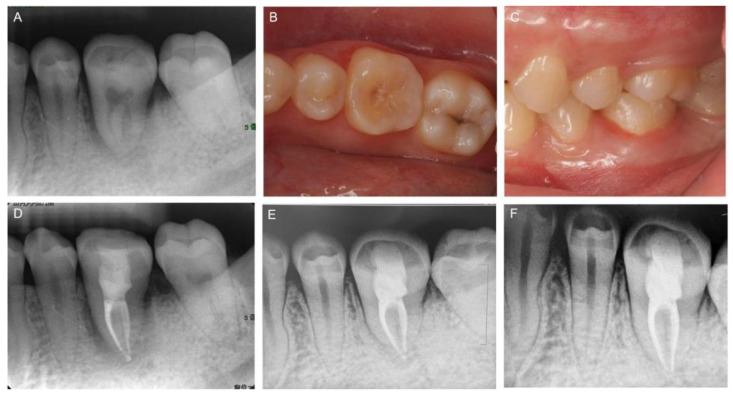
Clinical follow-up. (A) Postoperative radiograph taken immediately after autotransplantation. An occlusal view (B) and a buccal view (C) at the 3-week follow-up. (D) Endodontic treatment performed after 3 weeks. The 8-month follow-up radiograph (E) and 1-year follow-up radiograph (F) showing no signs of root resorption and the normal functioning of the transplanted tooth.
